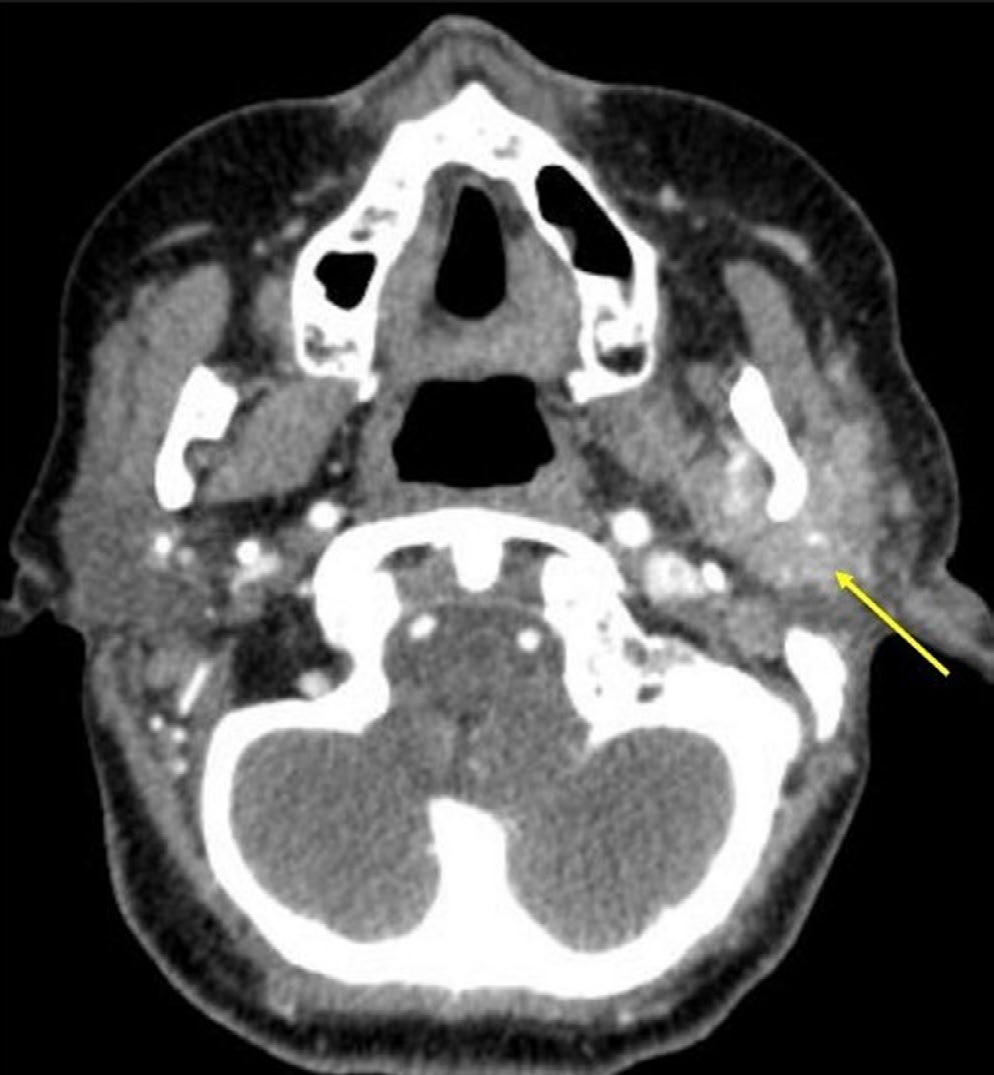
Axial CT scan with contrast. The arrow points toward the parotid lesion on the left side
